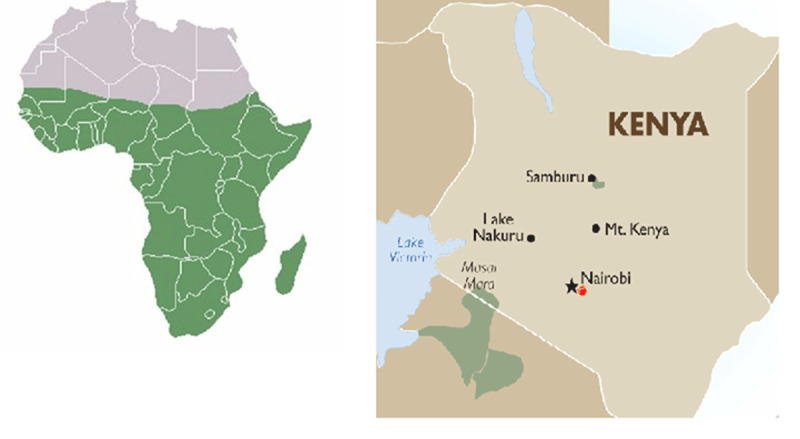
Map of Sub-Sahara Africa and Kenya. Map of Sub-Sahara Africa and Kenya. The Aga Khan University Hospital is a private 300 bed referral Centre located in Nairobi, Kenya’s capital city, receiving patients from the Aga khan outreach clinics in East Africa and other hospital both private and public in the East African community, a conglomerate of 10 countries. It is equipped with various modalities of cardiac imaging; an echocardiography lab, CT coronary angiography, Cardiac MRI and ischemia testing. It has two operational catheterization laboratories and is accredited as a primary PCI Centre. It has a dedicated coronary care unit as well as a 5-day outpatient cardiac clinic for ambulatory patients. It serves a multiracial community.
